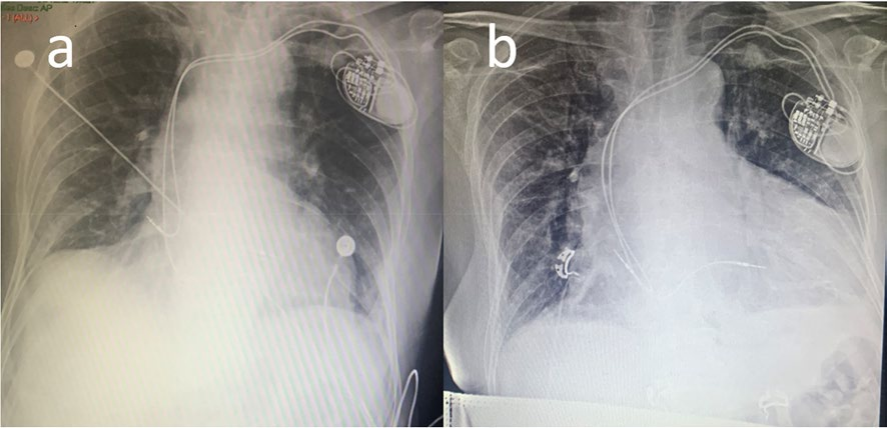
Chest X-ray (a: one day after pacemaker implantation, b: this time, two months after pacemaker implantation) b: Multiple patchy high-density shadows in both lungs, enlarged heart shadow, blurred left costophrenic angle
